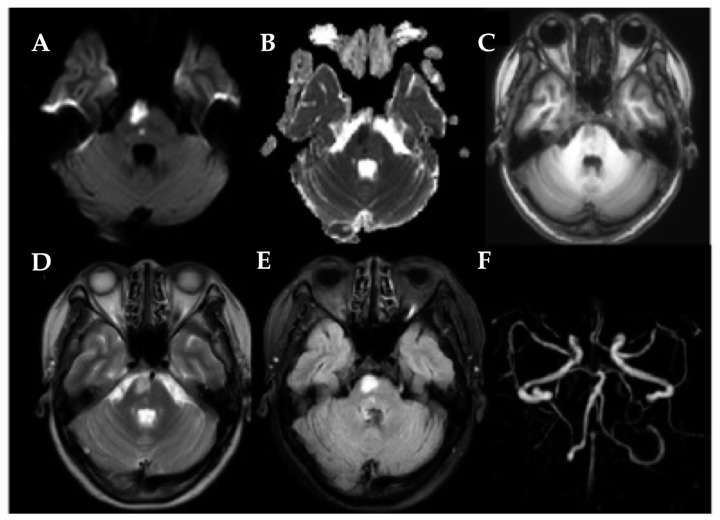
A 55-year-old female patient was referred for a right pontine infarction case. (A) High signal on DWI axial images; (B) low signal on ADC; (C) low signal on T1-weighted section; (D) high signal on T2-weighted section; (E) high signal on FLAIR; (F) no evidence of vascular occlusion on the MRA 3D-TOF.
